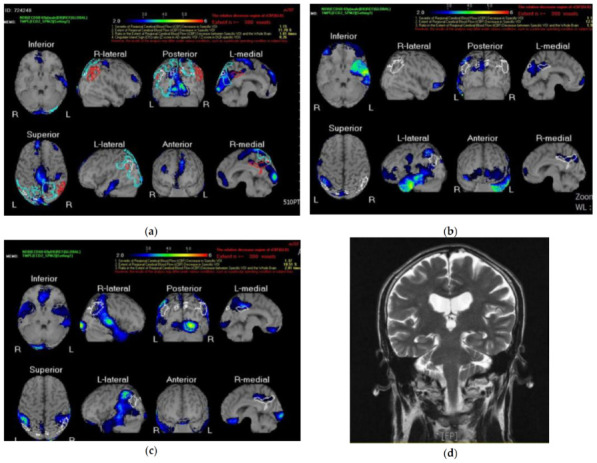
MRI/SPECT scans of the selected participants diagnosed with (a) bvFTD (participant no. 1), (b) nfvPPA (participant no. 7), (c) nfvPPA with atypical perfusion scan (participant no. 8), and (d) MCI/AD (participant no. 2). For (a–c), the perfusion SPECT images were evaluated using automated *z*-score surface mapping analysis, where the blue (red) color on the color scale represents mild (severe) disease, while for (d), hippocampus atrophy was determined using MRI-based hippocampal volume measurements. For *z*-score maps, the “Japanese/Chinese” gender- and age-matched database of normal brains was used to avoid age-related physiological changes in the regional cerebral blood flow.
